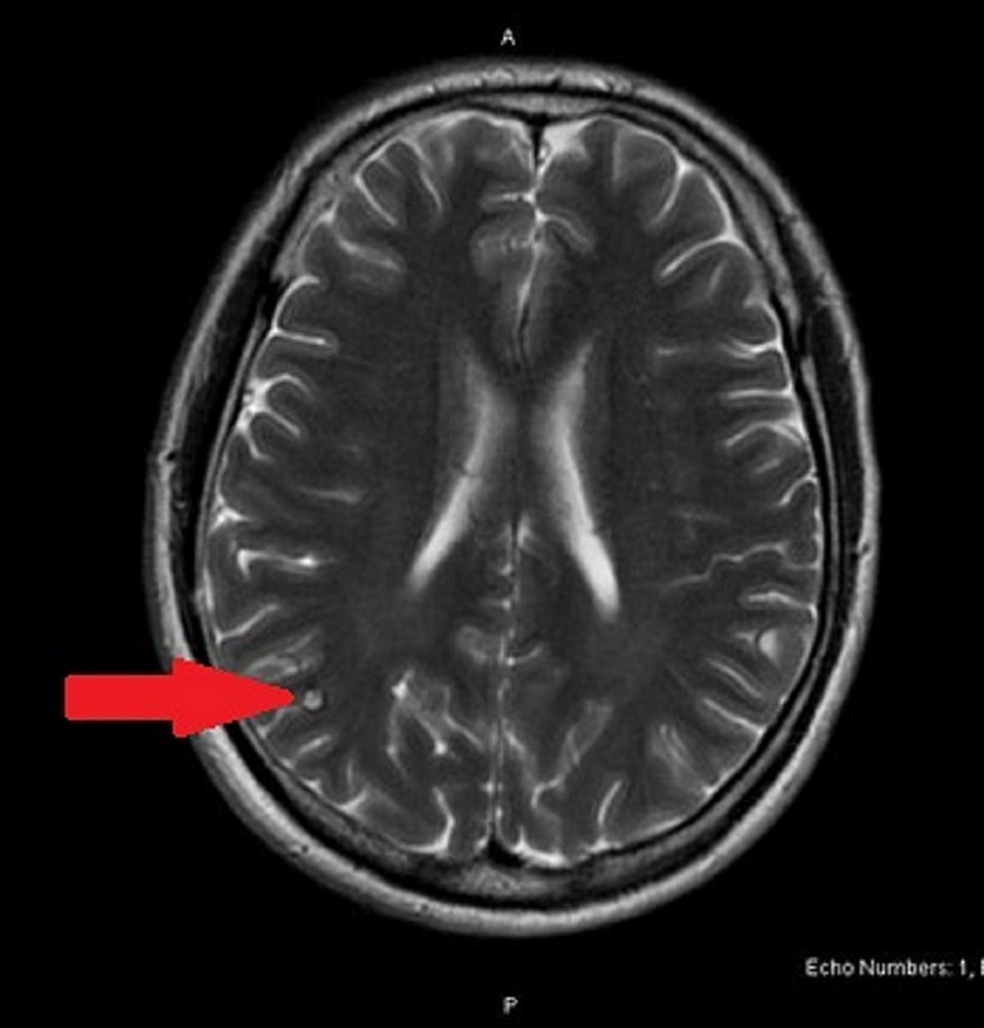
MRI of the brain with contrast showing a 5-mm T2 hyperintense, homogeneously enhancing lesion in the right parietal lobe with associated calcification.
